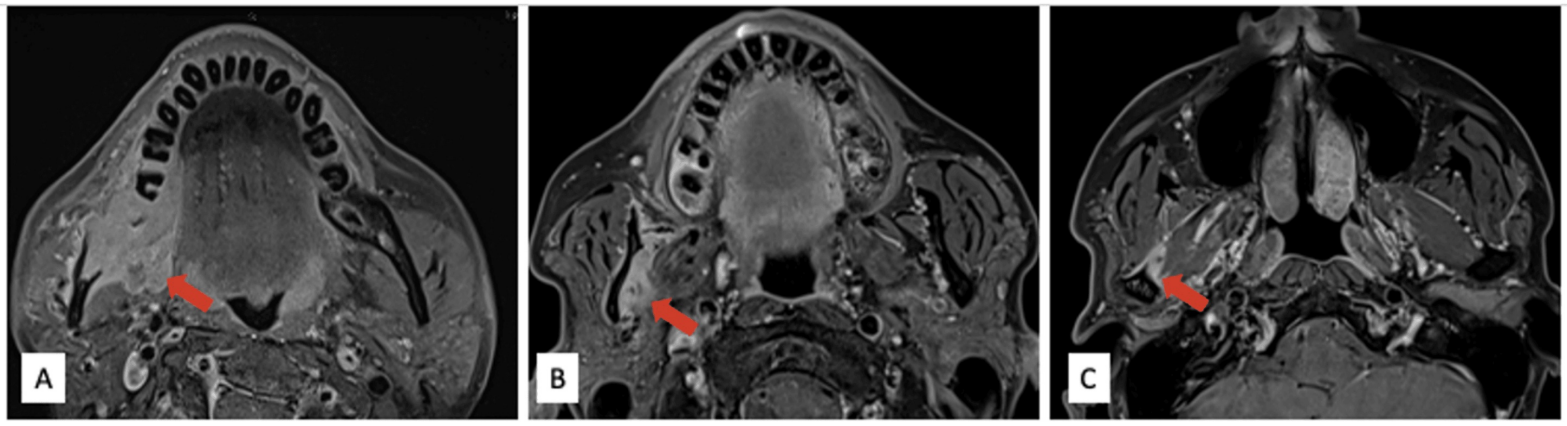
MRI neck axial T1 fat-saturated (T1FS) post gadolinium images. Enhancing mass (red arrow) at the level of (A) the body of the right hemimandible extending to (B) the ramus of the mandible and (C) mandibular condyle with the involvement of adjacent medial and lateral pterygoid muscles.
